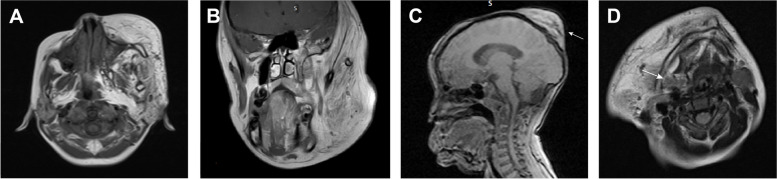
Maxillofacial imaging findings. A T1-weighted axial image of patient 30. The patient exhibited evident subcutaneous adipose tissue proliferation, with infiltration observed in the temporalis muscle, masseter muscle, and parotid gland, resulting in the loss of normal boundaries. B T1-weighted coronal image of patient 12. Extensive fatty tissue with wide-ranging borders infiltrated superiorly into the temporal muscle. C Sagittal image of patient 1. A soft tissue mass was observed on the affected side of the scalp (white arrow), displaying fat signal. D T2-weighted axial image of patient 18. The submandibular gland of the patient was also affected by fatty infiltration (white arrow)
